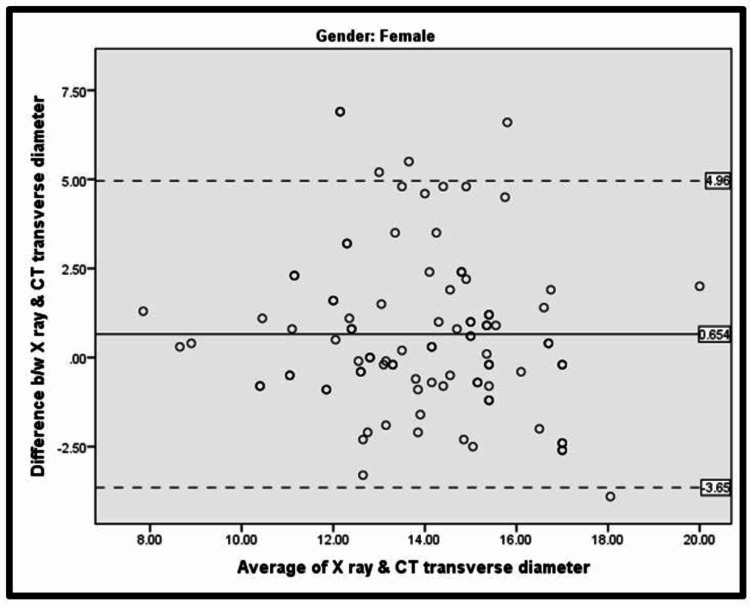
Bland-Altman plot for X-ray vs CT transverse diameter among females The solid line shows bias, and the dotted lines show the limit of agreement.
